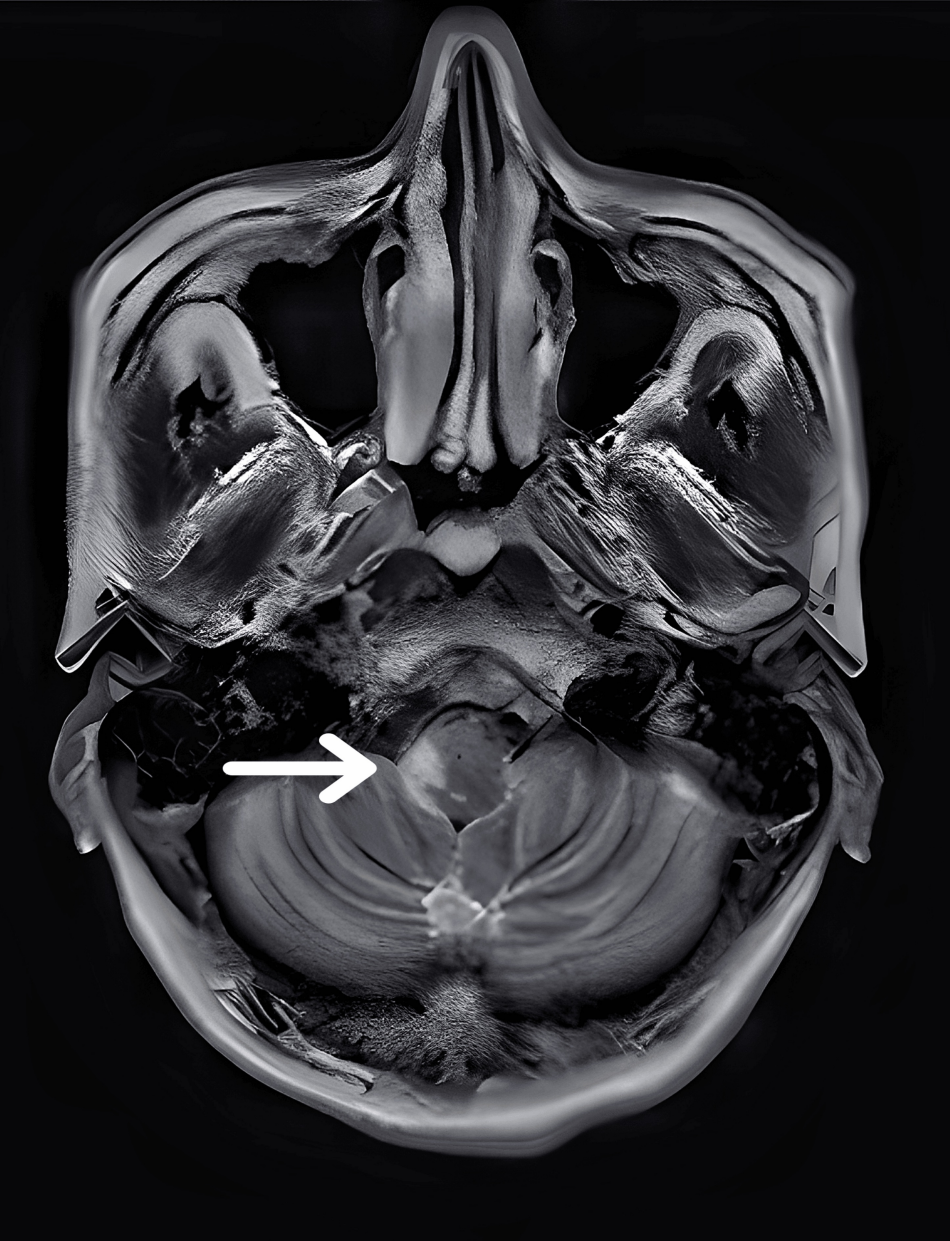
T2-weighted magnetic resonance imaging (MRI) demonstrating an infarction in the right lateral medulla
